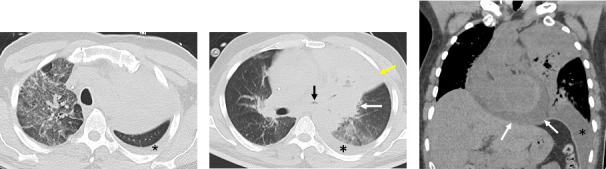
CT without contrast axial views 7 days later (a, b) in the lung window show worsening of left upper lobe consolidation (yellow arrow) and left perihilar consolidation (white arrow), diffuse narrowing of the left bronchi with heterogenous intraluminal material (black arrow), and right upper lobe and left lower lobe ground-glass opacities (reverse halo sign). Left pleural effusion is visualized (asterisk). Coronal soft tissue window view (c) shows a new pericardial effusion (white arrows) secondary to dissemination to the pericardium.
